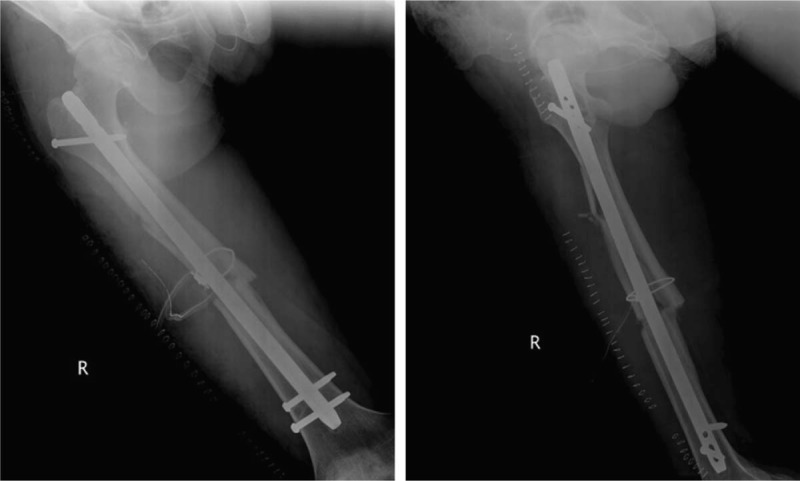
Radiographs after exchanging nailing using a new nail and a cerclage wire to fix the extended comminution of the fracture.
